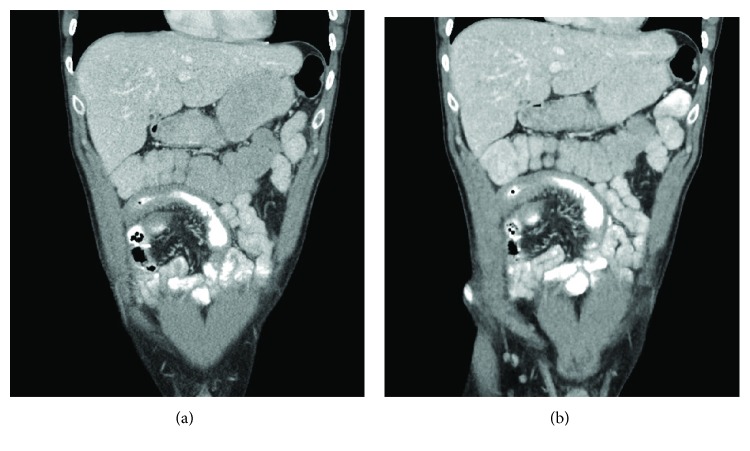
(a) Conventional dose CT reconstructed with ASIR and (b) low-dose CT reconstructed with MBIR demonstrating terminal ileum mural thickening and prominent vasa recta suggestive of acute inflammation. The low-dose CT entails an approximately 75% radiation dose reduction (reduced from 3.5 mSv to 0.98 mSv).
